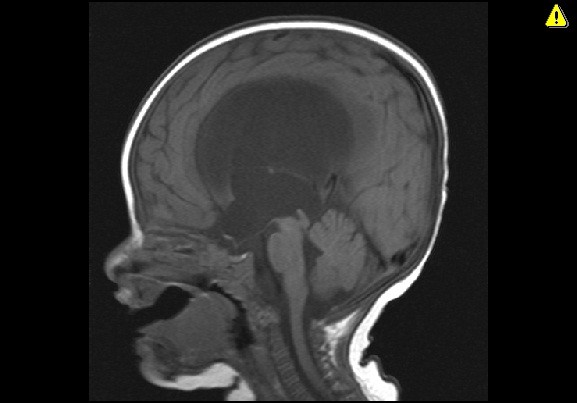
Sagittal T1-weighted magnetic resonance image demonstrating obstructive hydrocephalus due to congenital aqueductal stenosis.
